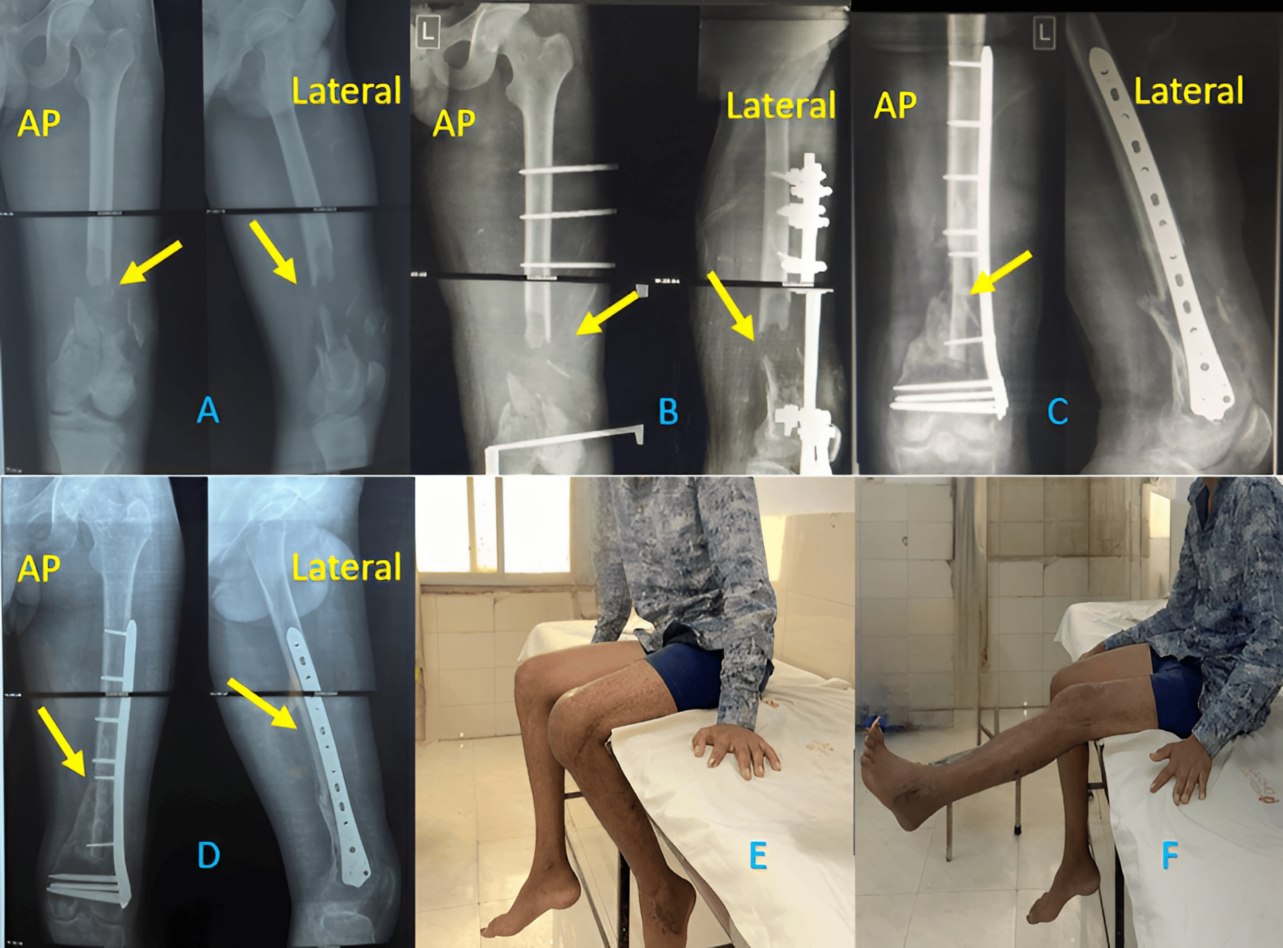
Results of DFLCP in open grade 3B fracture (A) AP and lateral views after the injury (arrow showing fracture), (B) AP and lateral X-rays after primary stabilization with an external fixator (arrow showing the fracture), (C) Post-operative X-rays after fixation with DFLCP and fibula strut graft (arrow showing the graft), (D) X-rays at the final follow-up (arrow showing well-united fracture), (E) Knee flexion at the final follow-up, and (F) Knee extension at the final follow-up DFLCP: Distal Femur Locking Compression Plate
